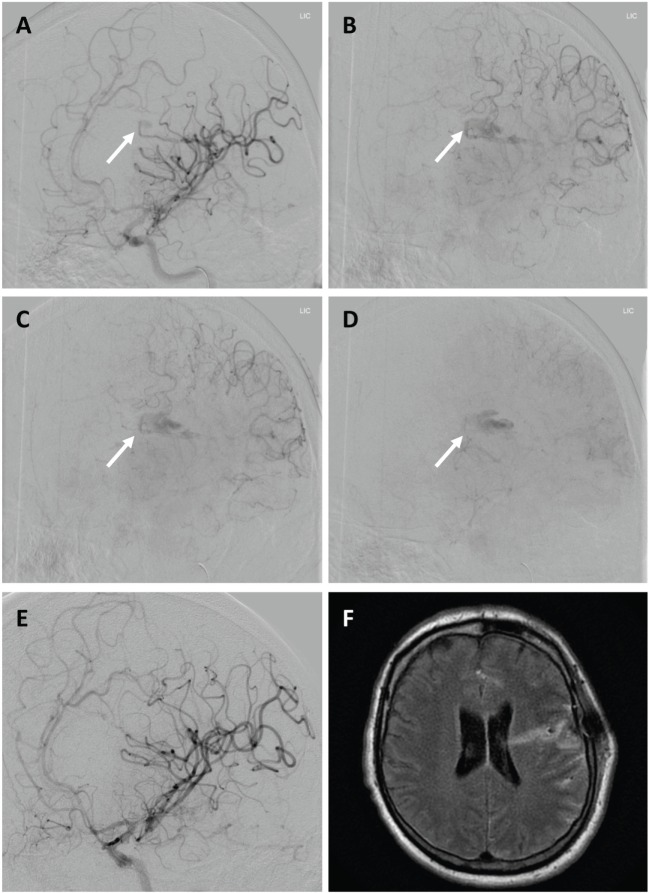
Ventricular tract hemorrhage following intracranial nail removal. (A–D) Serial oblique projections of a left internal carotid angiogram immediately after nail removal, demonstrating active hemorrhage (white arrows) from a small left M3 branch extending down the puncture tract into the lateral ventricle. (E) Lateral left internal carotid angiogram confirming resolution of hemorrhage after no active extravasation was seen on serial M3 microcatheterizations. No aneurysmal dilatation or flow-limiting stenosis is seen. (F) Axial T2-weighted flair brain MRI demonstrating only mild local inflammation 5 days after nail removal.
